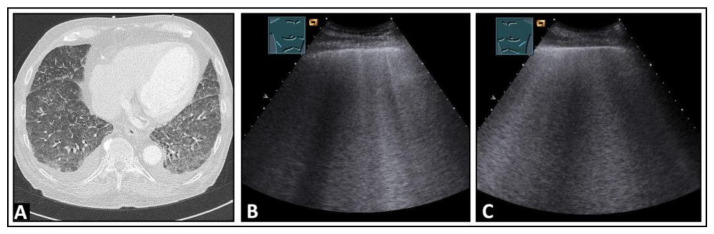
A patient with aortic valve insufficiency, progressive dyspnea, and cardiac pulmonary edema. (A) On chest computed tomography, bilateral pleural effusion and diffuse ground-glass opacities with septal thickening are visualized, consistent with congestion. (B,C) On lung ultrasound, increased B-lines are present bilaterally in the lower fields.
